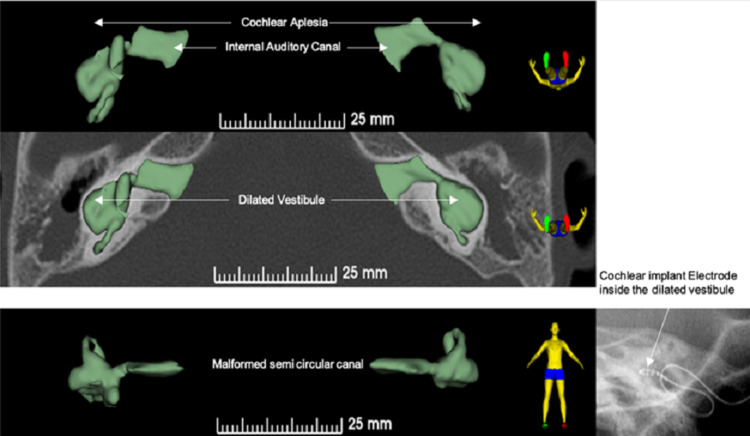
The preoperative planning using a 3D reconstruction for the CT findings of the studied case.
